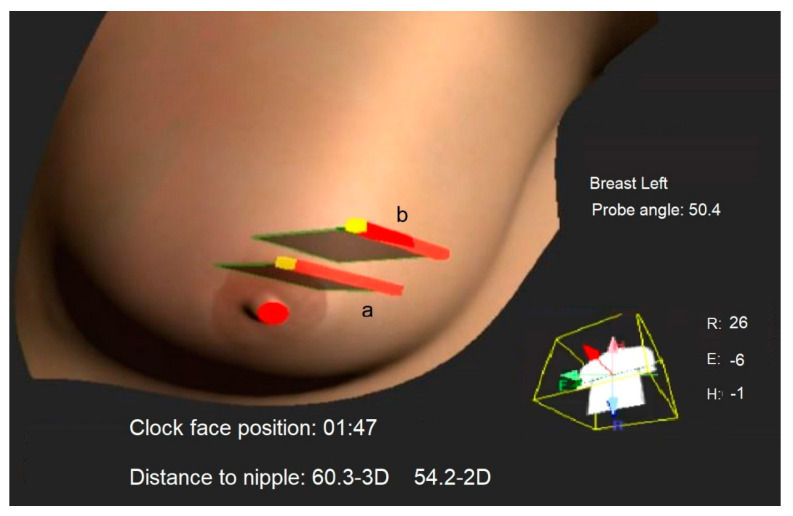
The 3D ultrasound frame position and orientation from a previous exam, labeled as ‘a’, is displayed as a gray rectangle. This is shown concurrently with the real-time ultrasound frame during the current exam, labeled as ‘b’. This approach assists consecutive operators in reproducing the 3D mass location by aligning frame ‘b’ with frame ‘a’. The probe head is represented by a red line, the orientation marker by a yellow dot and the nipple point by a red dot. Additionally, metrics about the current probe position relative to the nipple and body, as well as the body’s rotation on the exam table, are displayed in real-time for operator guidance.
